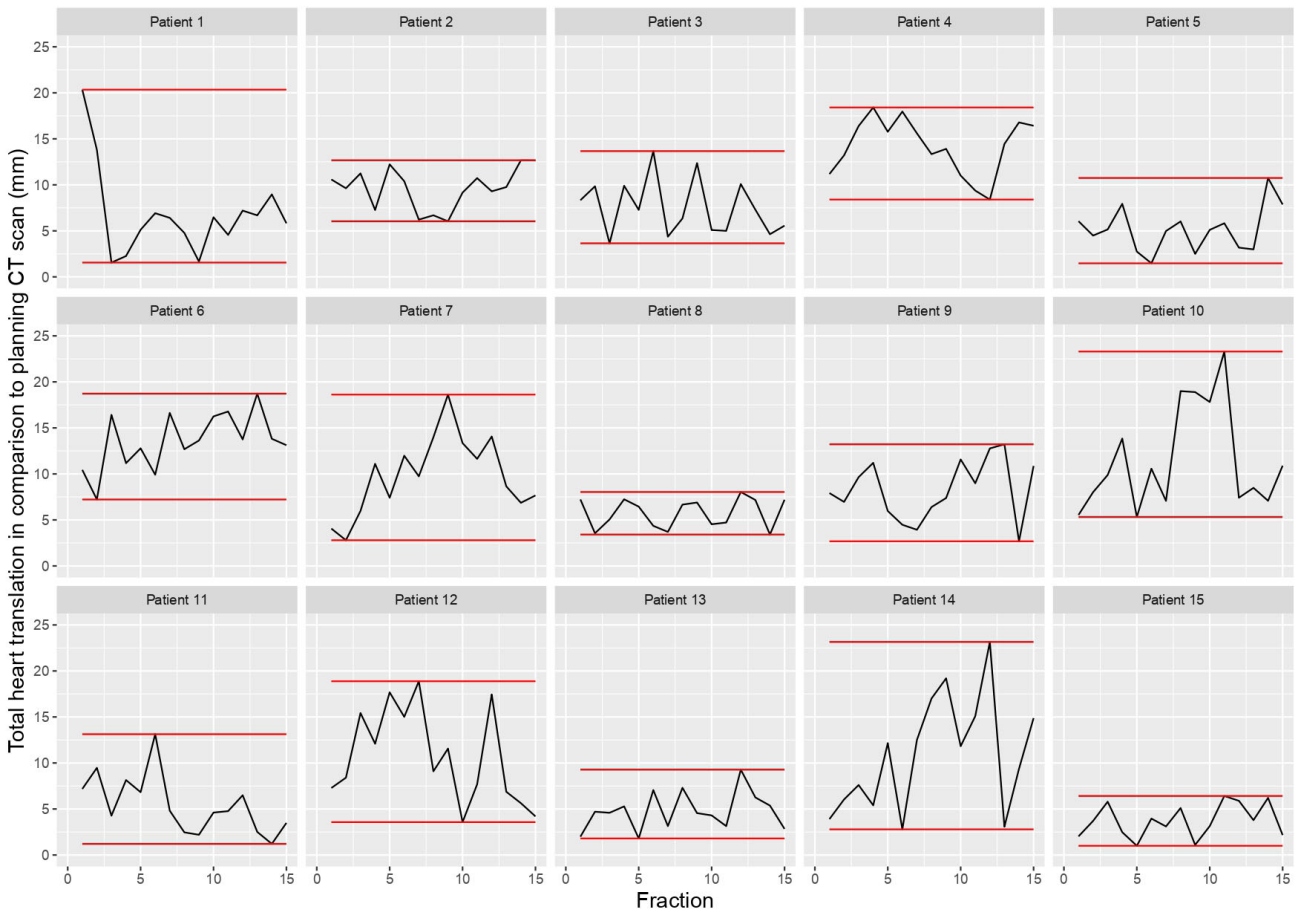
Individual 3D heart translation per patient (n = 15) in comparison to the planning CT scan, with the minimal and maximal translation (red line).
